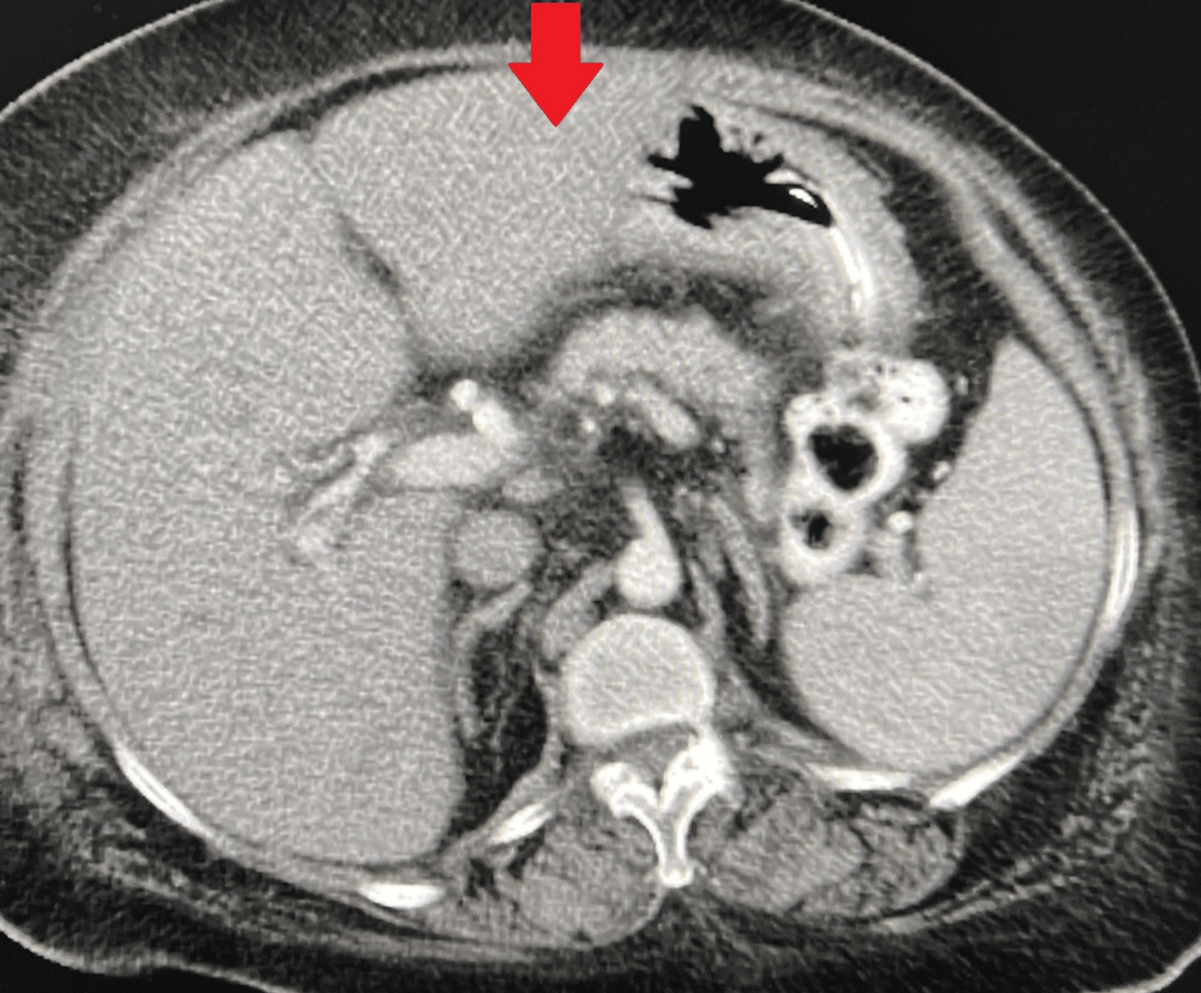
Follow-up axial CT obtained 48 hours later demonstrating complete resolution of HPVG. The red arrow indicates the same peripheral hepatic region previously involved, now without portal venous gas. HPVG: hepatic portal venous gas
